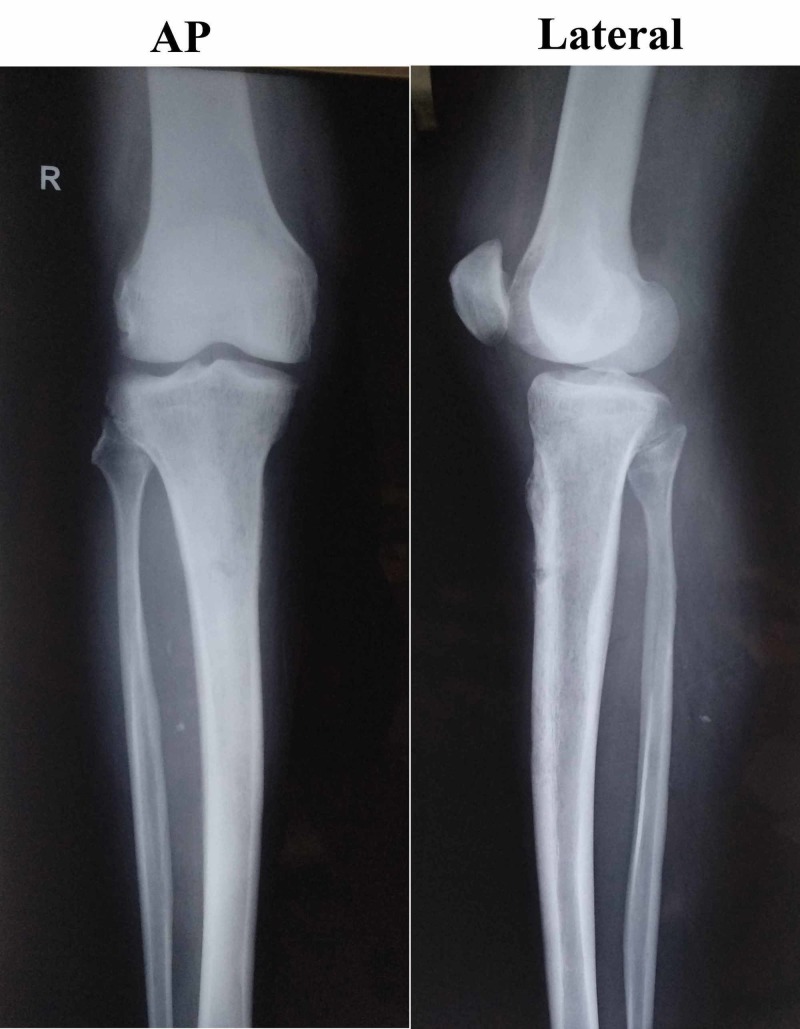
A one-month post-operative X-ray of the right knee joint with proximal leg and distal thigh, still not showing features of osteomyelitic changes.
